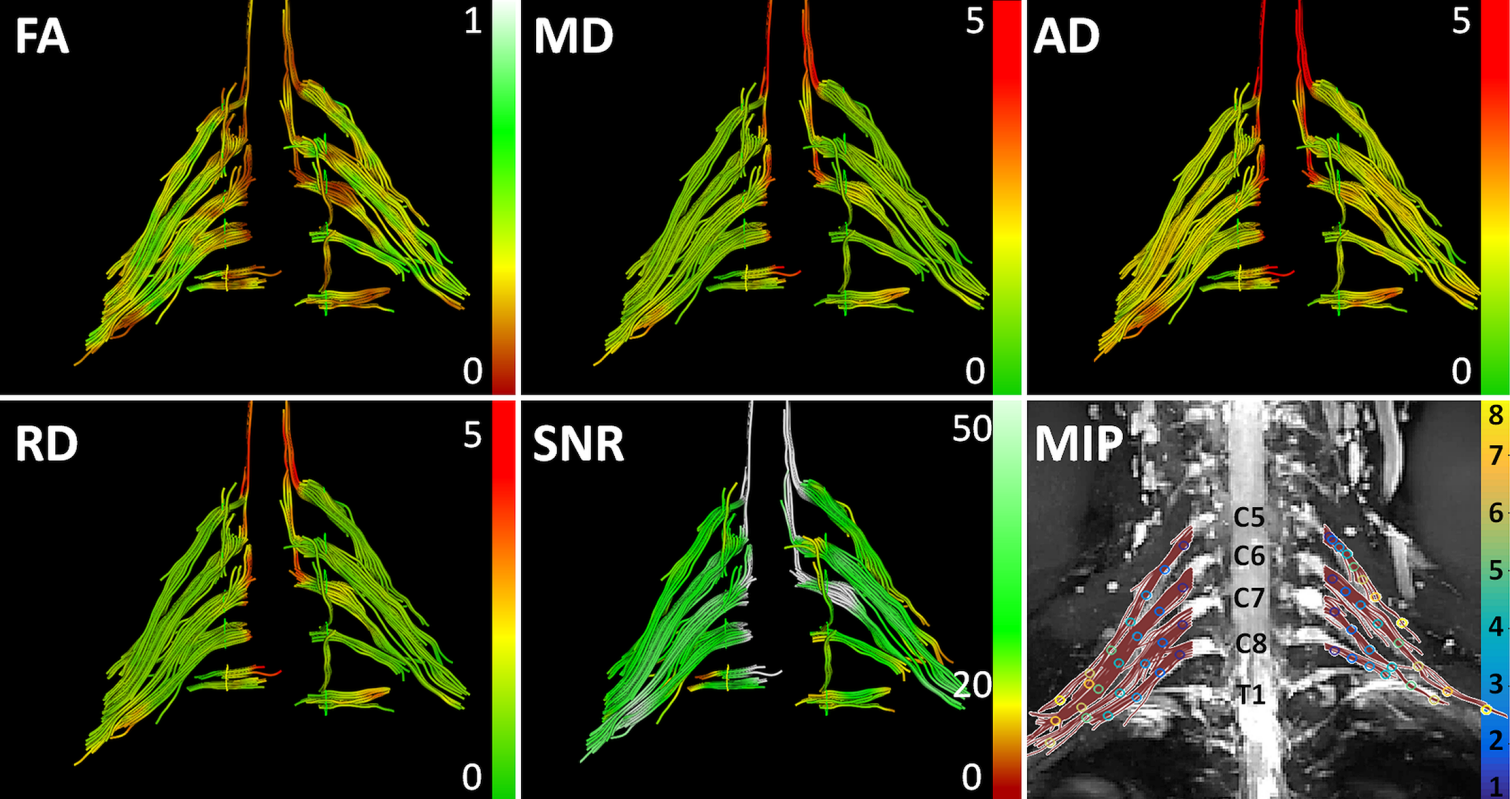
DT-MRI tractography. Representative example of a DT-MRI tractography dataset (64 years old male), including tractography color coded for fractional anisotropy (FA), mean diffusivity (MD), axial diffusivity (AD), radial diffusivity (RD) and signal to noise (SNR). The bottom right image is a maximum intensity projection (MIP) of the brachial plexus neurography image (MR-Neurography) with superimposed fiber tracts of the postganglionic tissue only. The circles along the tracts indicate the sample sections which are color-coded for sample number.
